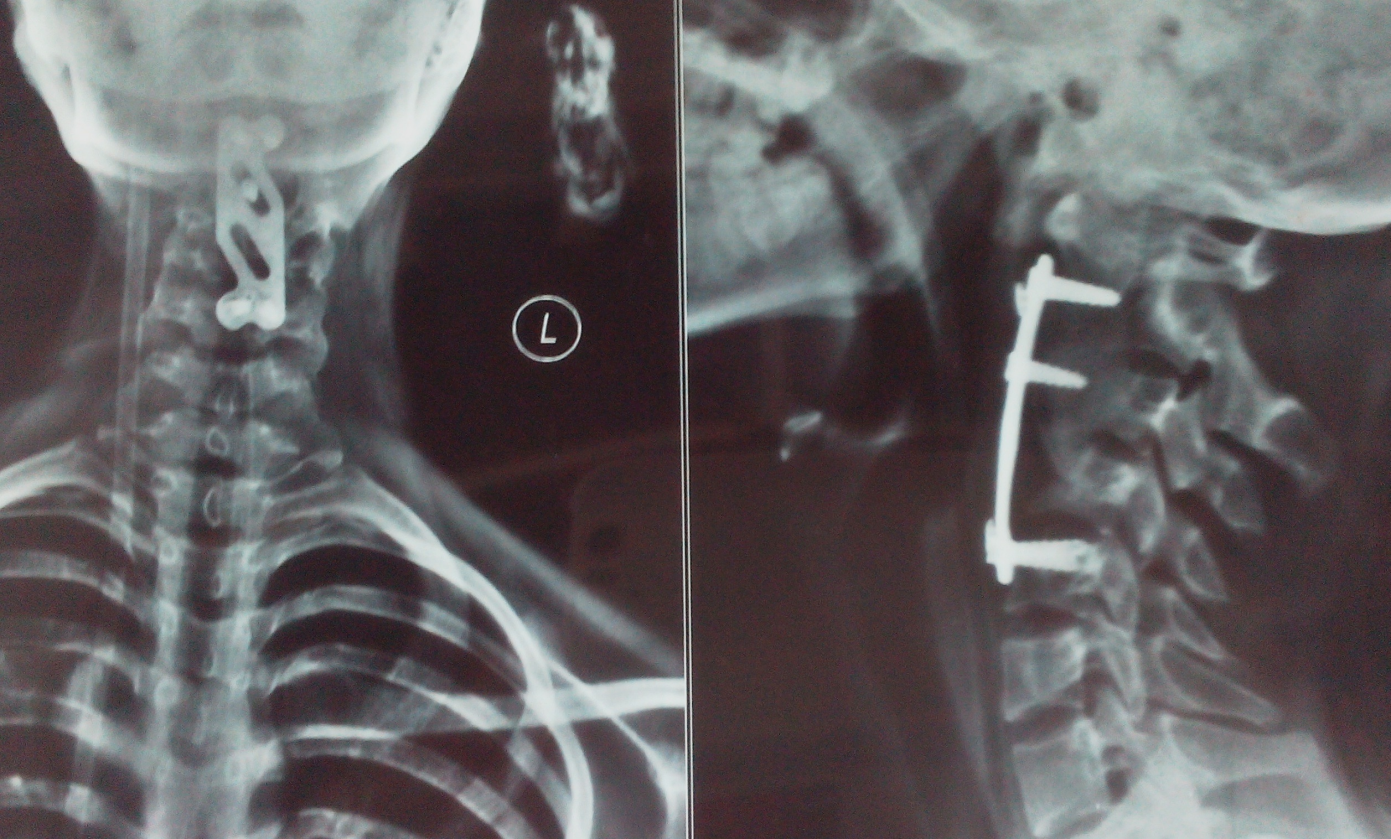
Postoperative X-rays, Anteroposterior View (left) and Lateral View (right) of the Cervical Spine Reconstruction of the odontoid process by a tricortical iliac crest graft and fixed with anterior cervical plate.
